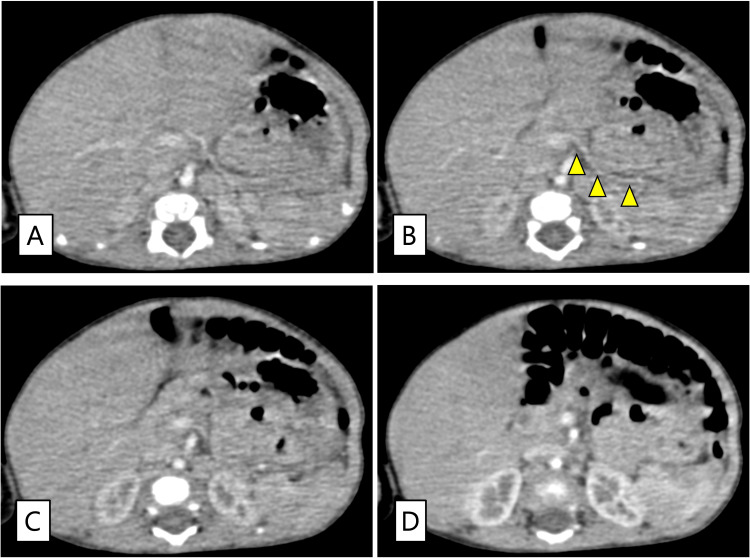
Abdominal contrast-enhanced computed tomography (CT). The images are 1-mm slice CT scans arranged in cranial-to-caudal order from panels A to D. The yellow arrowhead in panel B indicates the splenic artery. No pancreatic tissue can be observed ventral to the splenic vessels. The gallbladder is absent in the caudal portion of the right hepatic lobe, where it is typically located.
